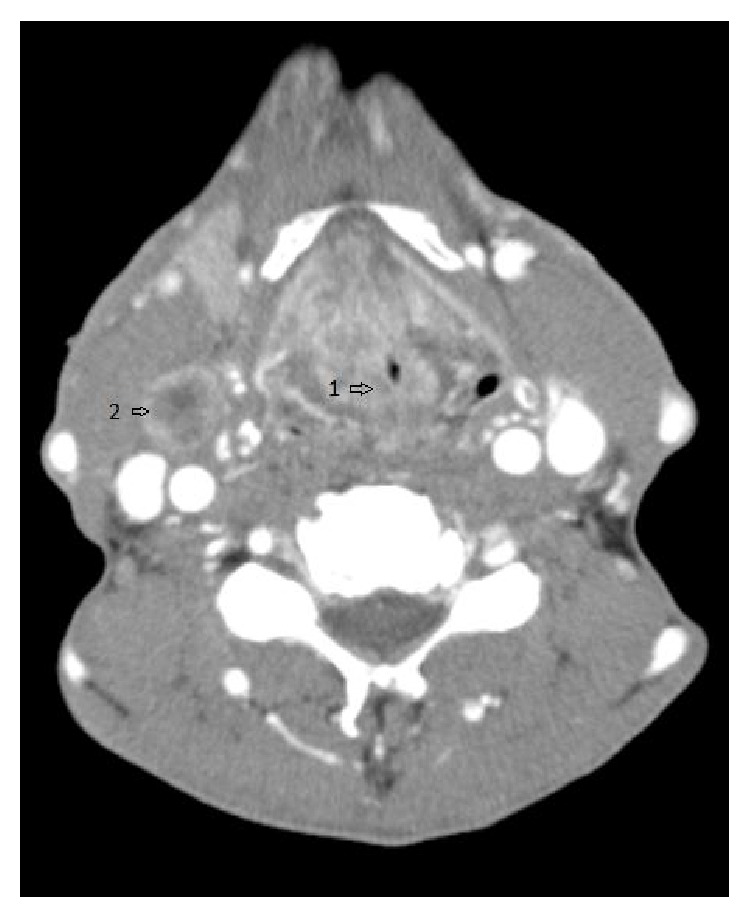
Supraglottic-level CT showing airway narrowed by glottis mass (1) and lymph node metastasis (2).
